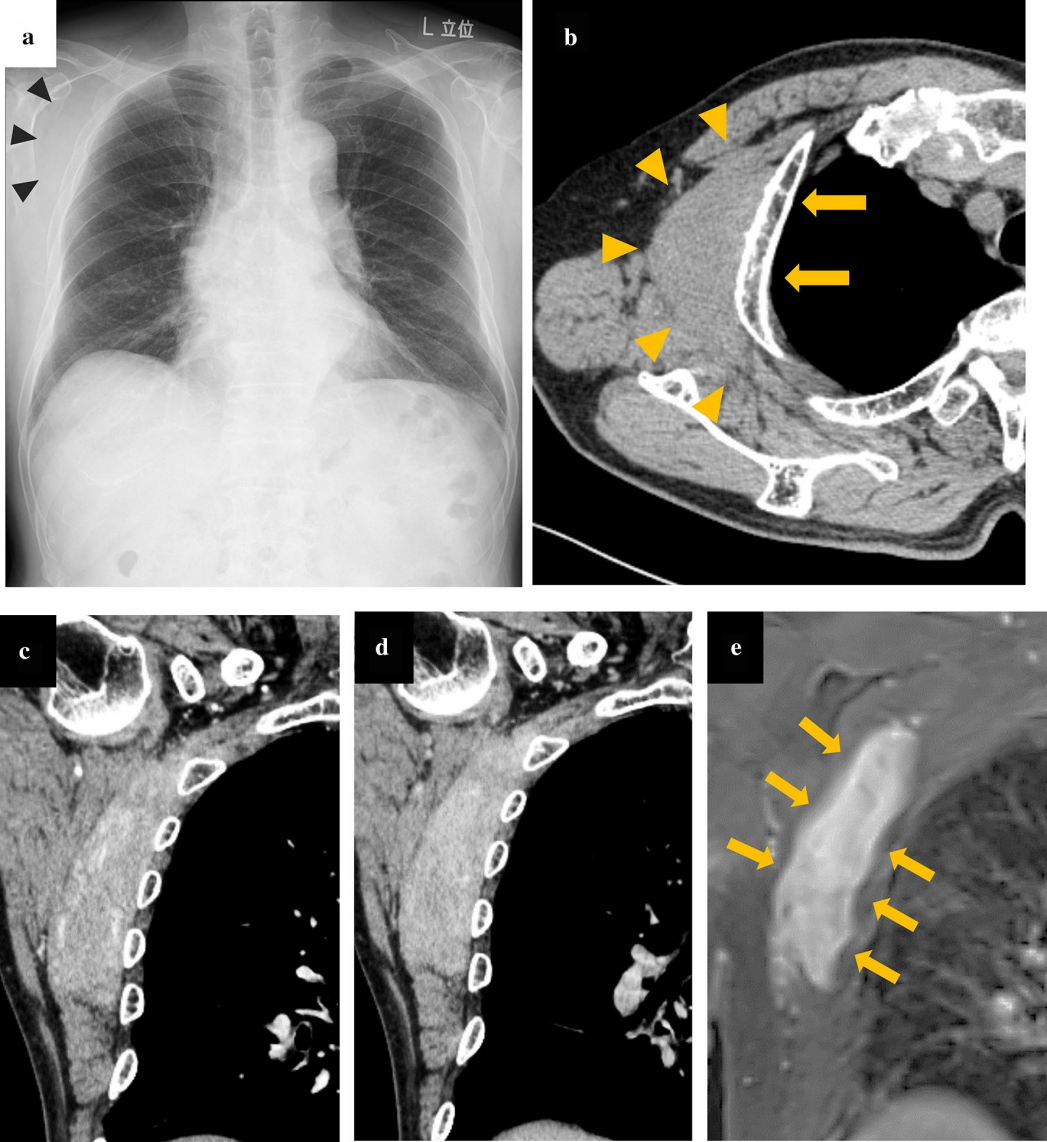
Radiological imaging of a case of desmoplastic fibroblastoma in the chest wall. a Chest radiograph demonstrating soft tissue thickening at the right lateral upper chest wall (arrowhead). b Axial view of a non-contrast computed tomography (CT) scan demonstrating the location of the tumor (arrowhead) and cortical bone erosion of the second rib (arrow). c, d Coronal view of a dynamic contrast-enhanced CT scan demonstrating the line of abundant vascularity at the periphery of the tumor during the early phase (c) and homogeneous enhancement during the delayed phase (d). e Coronal view of gadolinium-enhanced T1-weighted magnetic resonance imaging (MRI) with fat suppression, demonstrating inhomogeneous enhancement in the tumor, as well as rim-like contrast enhancement with uniform thickness at the tumor periphery (arrow)
